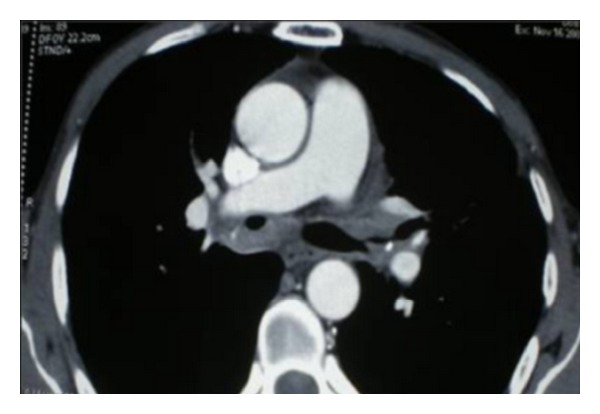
Computed tomography of the chest showing significant tracheobronchial wall thickening with narrowing of intermediate trunk.
